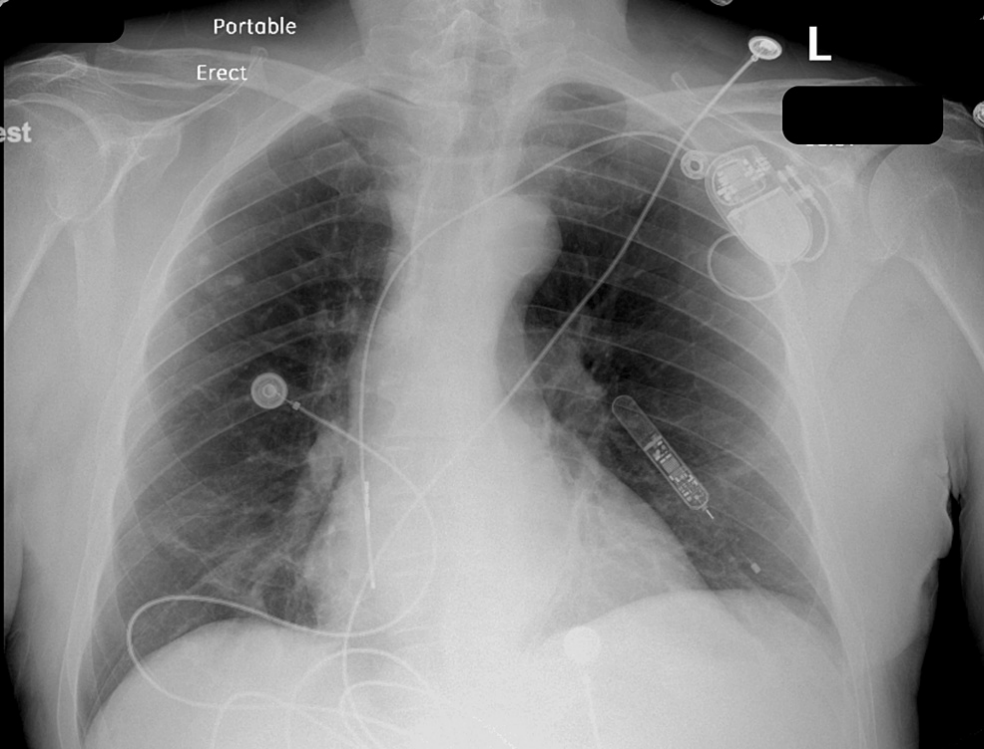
Chest X-ray after Right Ventricular lead removal Chest X-ray PA view shows the right atrial pacemaker lead in place.
